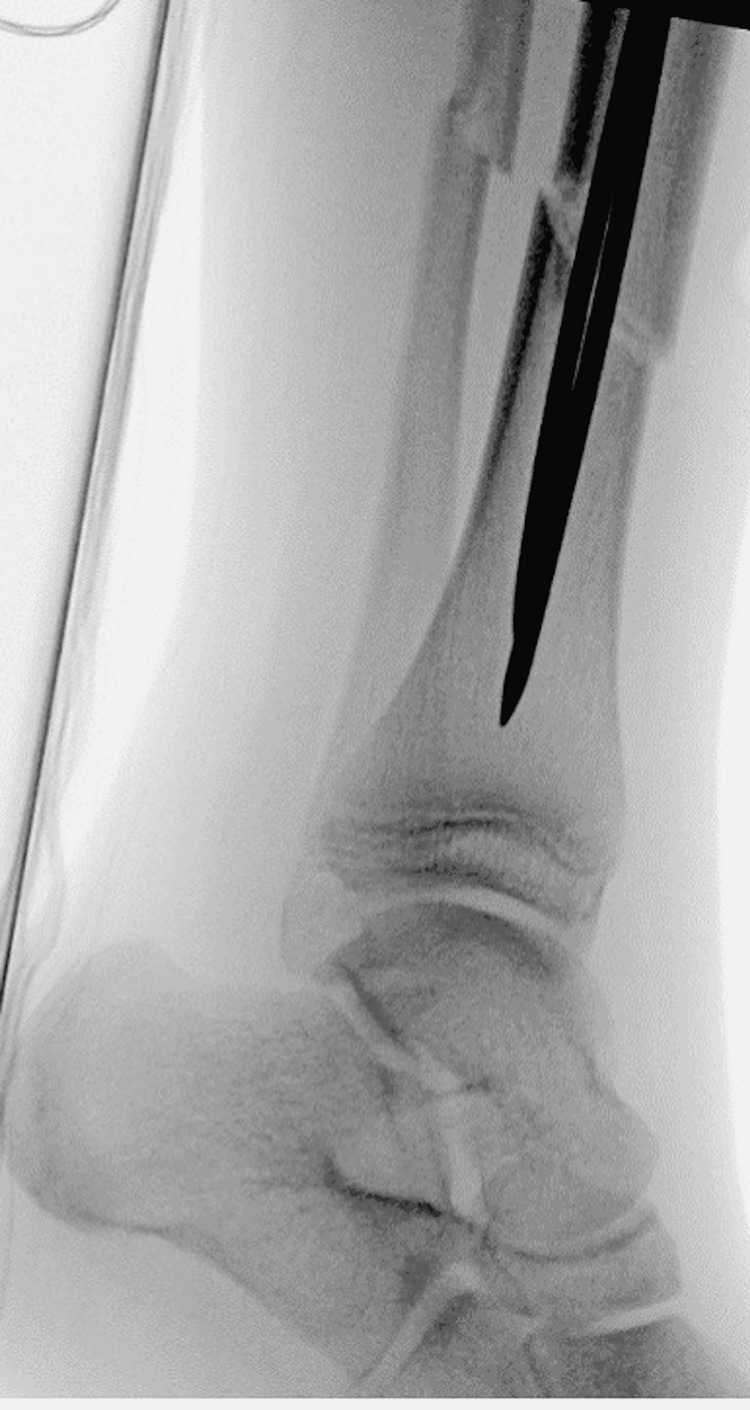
Lateral tibia-fibula intra-operative radiographs of irrigation and debridement with concomitant flexible nailing of the tibial shaft.
